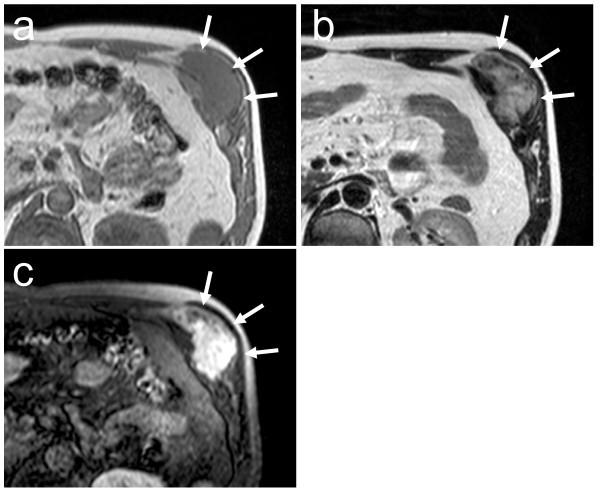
Axial MRI of the chest wall at his first visit. T1-weighted image (a) shows isosignal intensity area, whereas T2-weighted image (b) shows high intensity area. The tumor is strongly enhanced on gadolinium-enhanced T1-weighted image (c). Arrows indicate the tumor.
